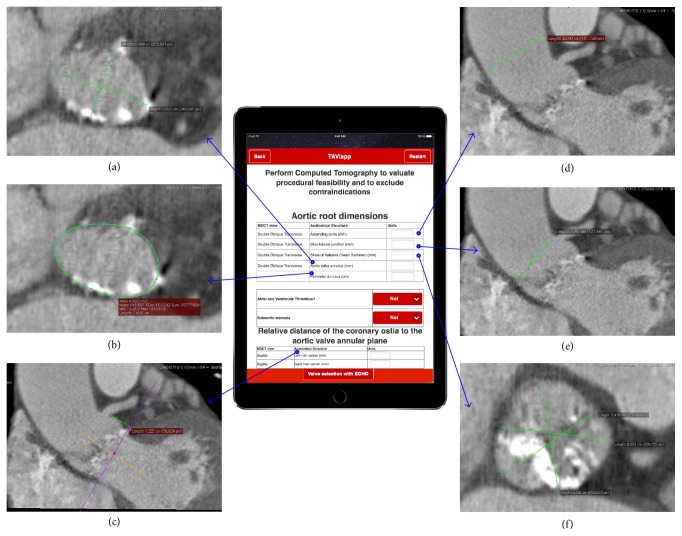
Selection of prosthesis size with TAVIApp using CT scan results. The figure reports a screenshot from TAVIApp (center) where the user can input the following results of CT scan measurements to be used for the selection of the most appropriate prosthesis size: (a) aortic valve annulus diameter; (b) aortic valve perimeter; (c) distance between the coronary ostia and the aortic valvular plane; (d) diameter of the ascending aorta; (e) aortic diameter at the sinotubular junction; (f) aortic diameter at the valsalva sinuses.
